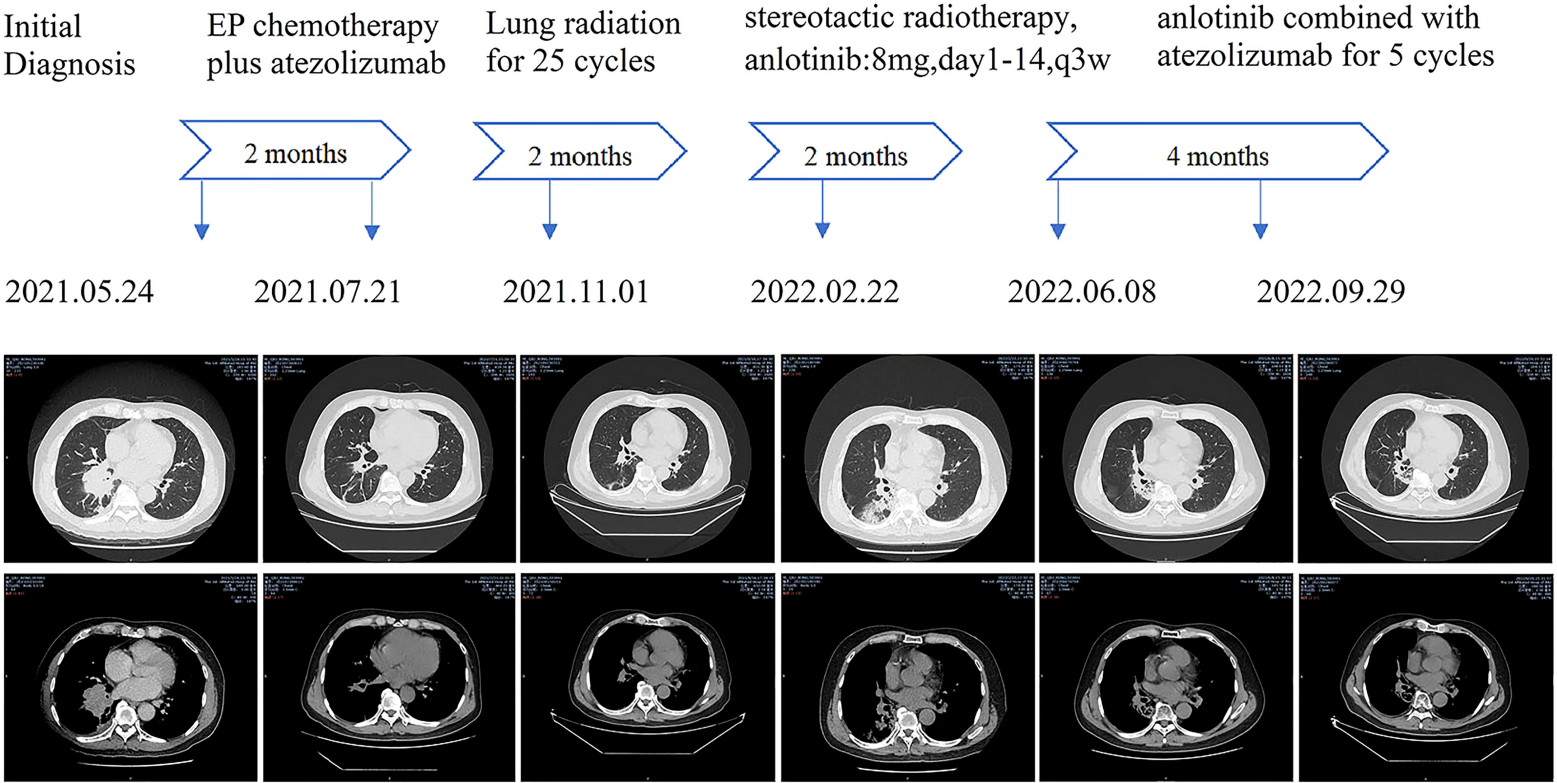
Chest CT images of primary lung noules at different time points.
